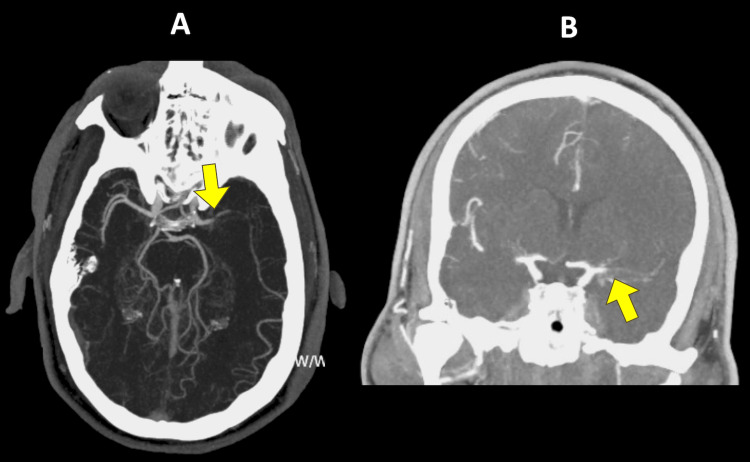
Missed identification by Viz LVO A 47-year-old male patient presented with a left M1 MCA occlusion after motor vehicle collision. The admission NIHSS score was 21. He was successfully revascularized with thrombectomy. The 24-hour post-intervention NIHSS score was 12. A) Viz LVO identification of M1 occlusion (arrow) on axial view of CT angiography helical reconstruction. B) Coronal view of non-contrast CT angiography with M1 occlusion (arrow). LVO, large vessel occlusion; MCA, middle cerebral artery; M1, M1 segment of the middle cerebral artery; NIHSS, National Institutes of Health Stroke Scale; CT, computed tomography.
